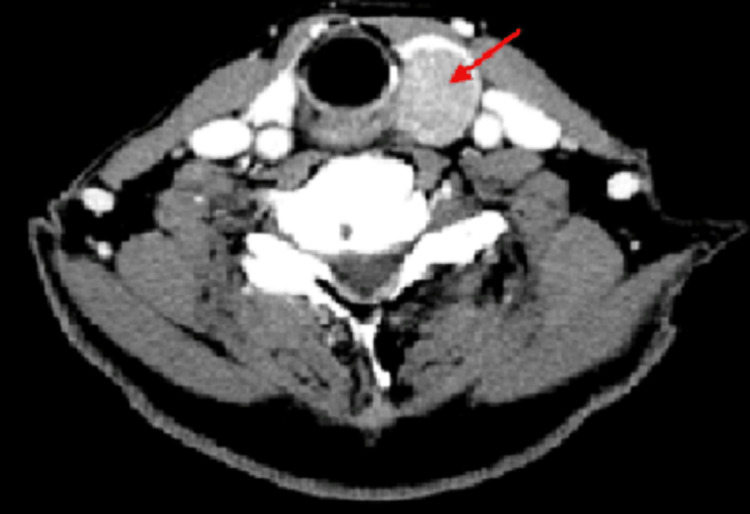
CT examination CT of the neck showed a tumor located on the posterior aspect of the left thyroid lobe. Tumor indicated by the red arrow.
